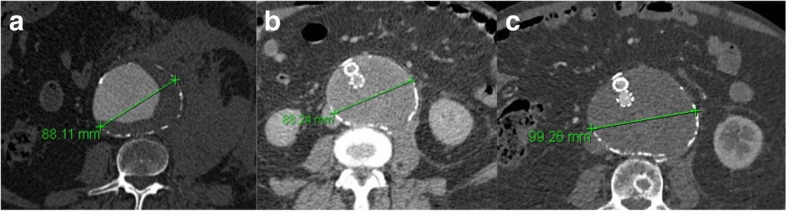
a-c CTA scan showing continuous expansion of the aneurysmal sac after initial repair for rupture. The expansion occurred over three years reaching a size of nearly 10 cm but without any evidence of an endoleak. It was considered as a result of “endotension”
